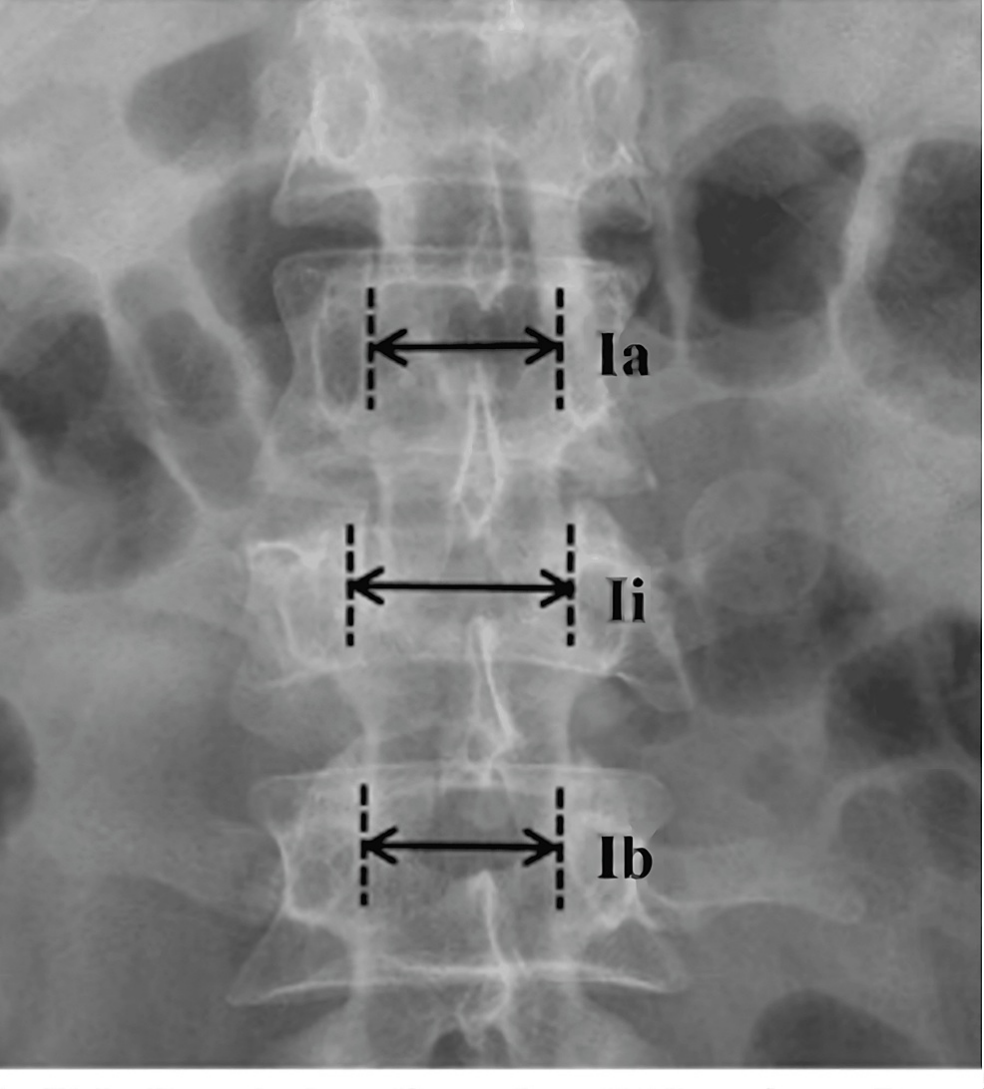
Interpedicular distance (IPD) is measured from an anteroposterior radiograph. It is measured from the medial sclerotic area of the pedicles at the level of fracture (1i). Normal IPD is defined as the average (In) of the corresponding distance above (1a) and below (1b) the injured level and calculated as (1a+1b)/2.
